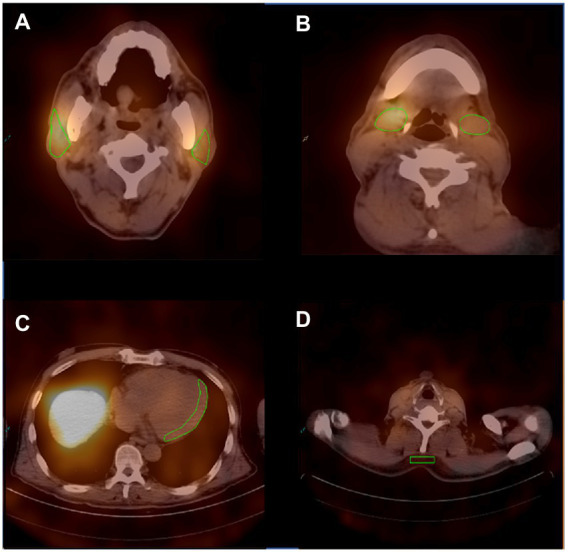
Representative ROIs of MIBG scintigraphy. (A) The ROIs of the bilateral parotid glands. (B) The ROIs of the bilateral submandibular glands. (C) The ROI of the left ventricular wall. (D) The ROI of the neck subcutaneous tissue.
